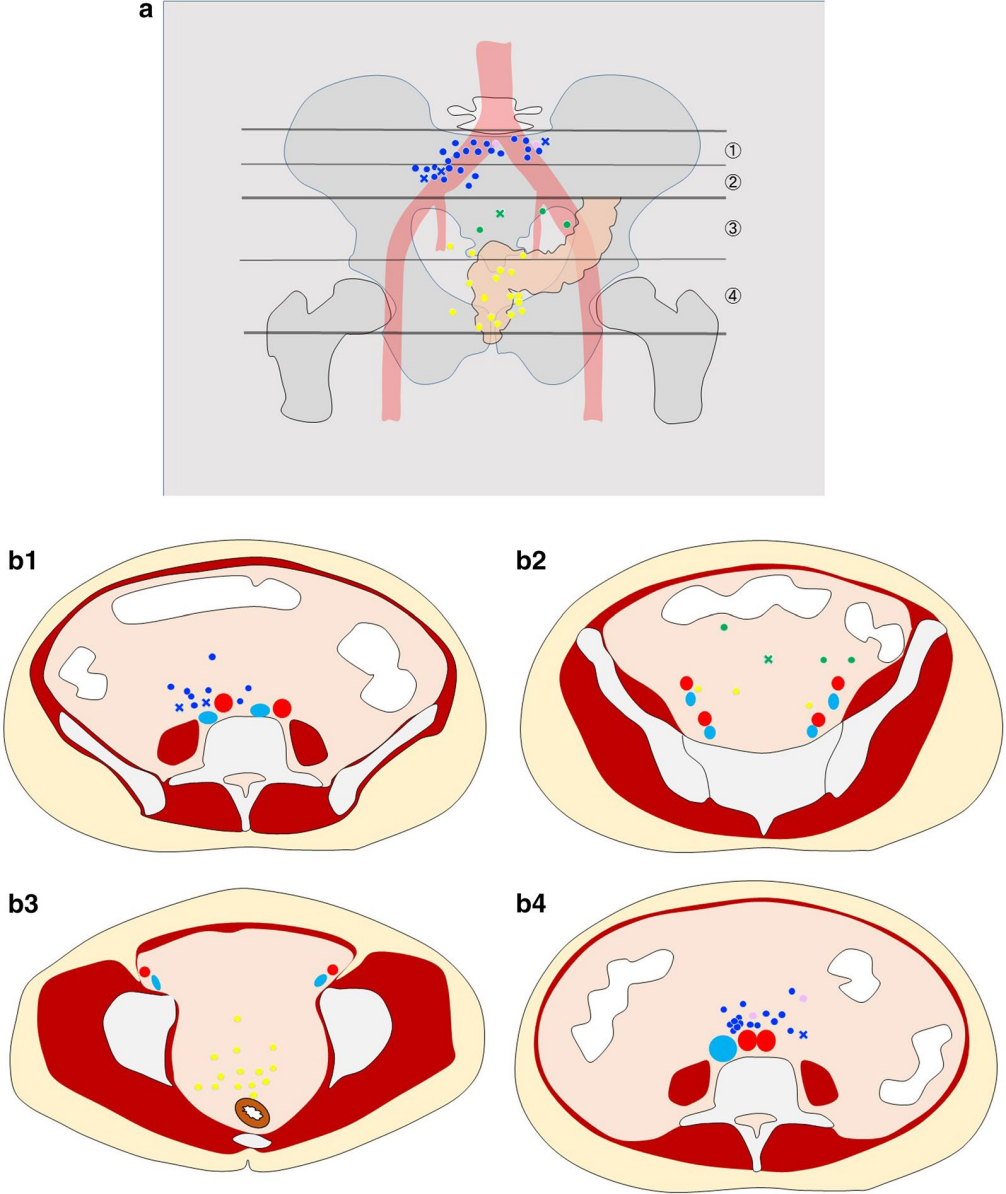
The location of the lesions, access route, and feasibility of endoscopic ultrasound-guided fine-needle aspiration. A The pelvic cavity was divided into four levels—the aortoiliac bifurcation, internal–external iliac artery bifurcation, sigmoid colon, and rectum. Circle mark indicates successful puncture, although cross mark is unsuccessful puncture. Color indicates puncture sight as following: blue, the second portion of the duodenum; pink, stomach; green, sigmoid colon; yellow, rectum. B1 Axial image of the aorto-iliac bifurcation level. B2 Axial image of the internal–external iliac artery bifurcation level. B3 Axial image of the sigmoid colon level. B4 Axial image of the rectum level
